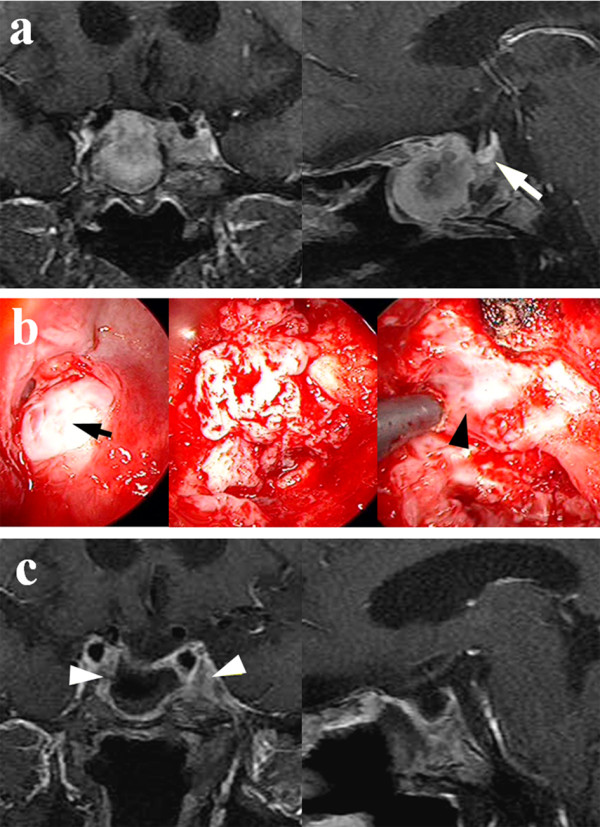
Before and after the first tumor resection. A. MRI shows an enhanced mass in the sphenoid sinus. The tumor extends into both cavernous sinuses surrounding internal carotid arteries. The pituitary gland and stalk are clearly identified (white arrow), and there is no tumor invasion in the sellaturcica. B. The photo shows a trans-nasal endoscopic view of the tumor. The anterior wall of sphenoid sinus is absent, and a whitish tumor (black arrow) is seen through the nasal cavity. The tumor was elastic and bled easily. There is no tumor invasion in the dura mater in the sellaturcica (black arrowhead). C. The tumor in the sphenoid sinus was totally removed; however, residual tumor is noted in cavernous sinuses (white arrowheads).
